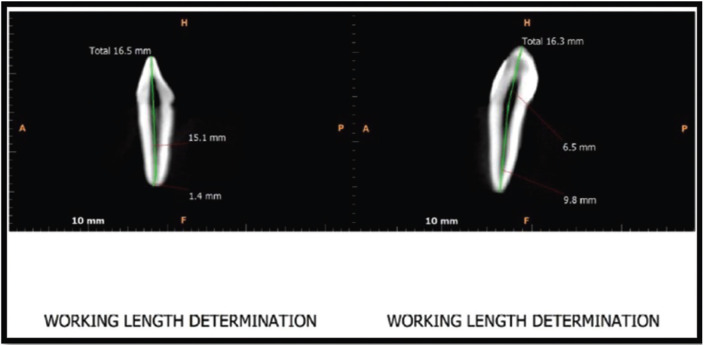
Teeth length measured with the help of CBCT.
